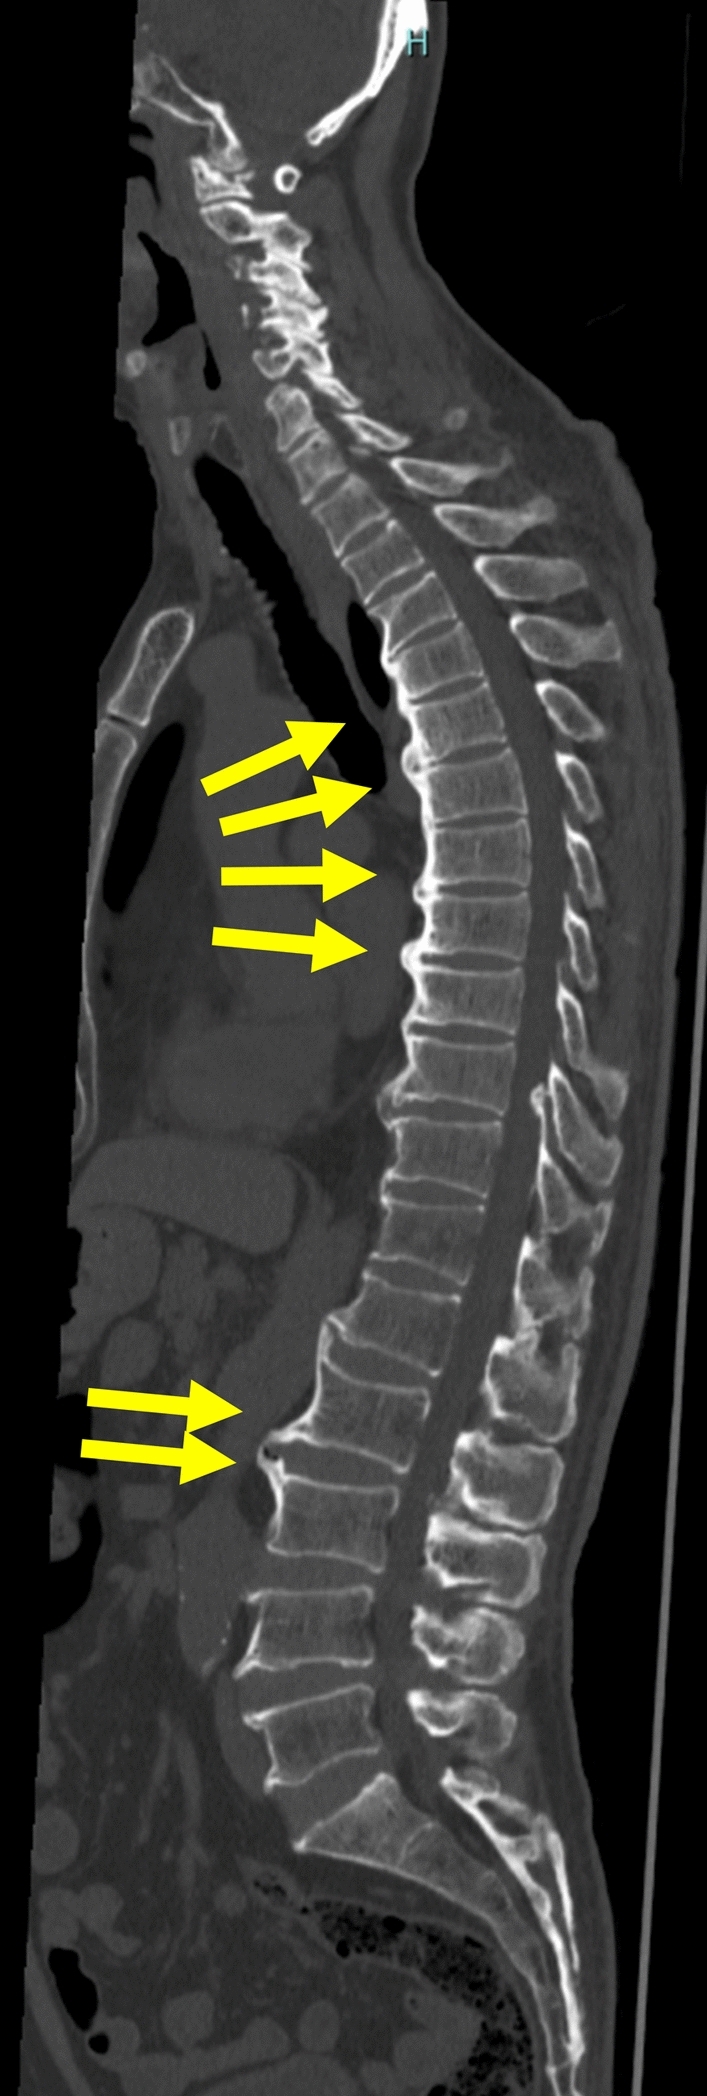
Ossification of the spinal ligaments in a patient with AIO. CT scan of the spine performed 39 years after disease onset in a patient with AIO shows ossification of the anterior longitudinal ligaments (arrows)
